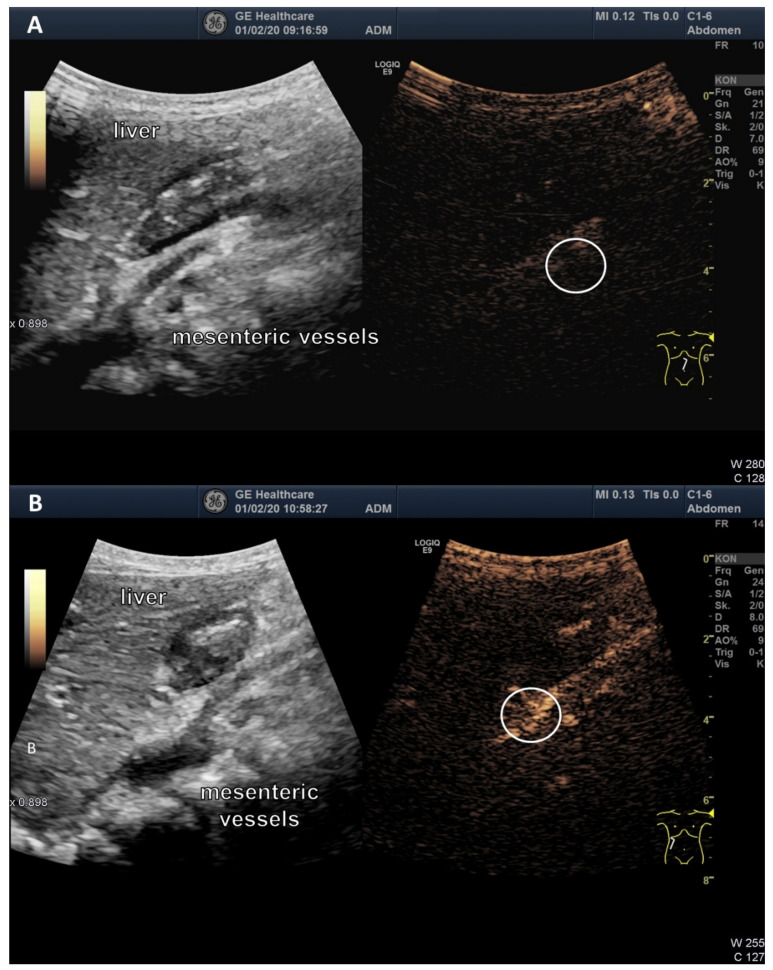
High-resolution ultrasound, multi-frequency C1-6 MHz probe. Abdominal ultrasound images before (A) and 90 minutes after oral application of vitamin C formula (B) from one volunteer. The circles show the mesenteric vessels. There is a clear microbubble signal indicating the intestinal absorption of liposomes.
